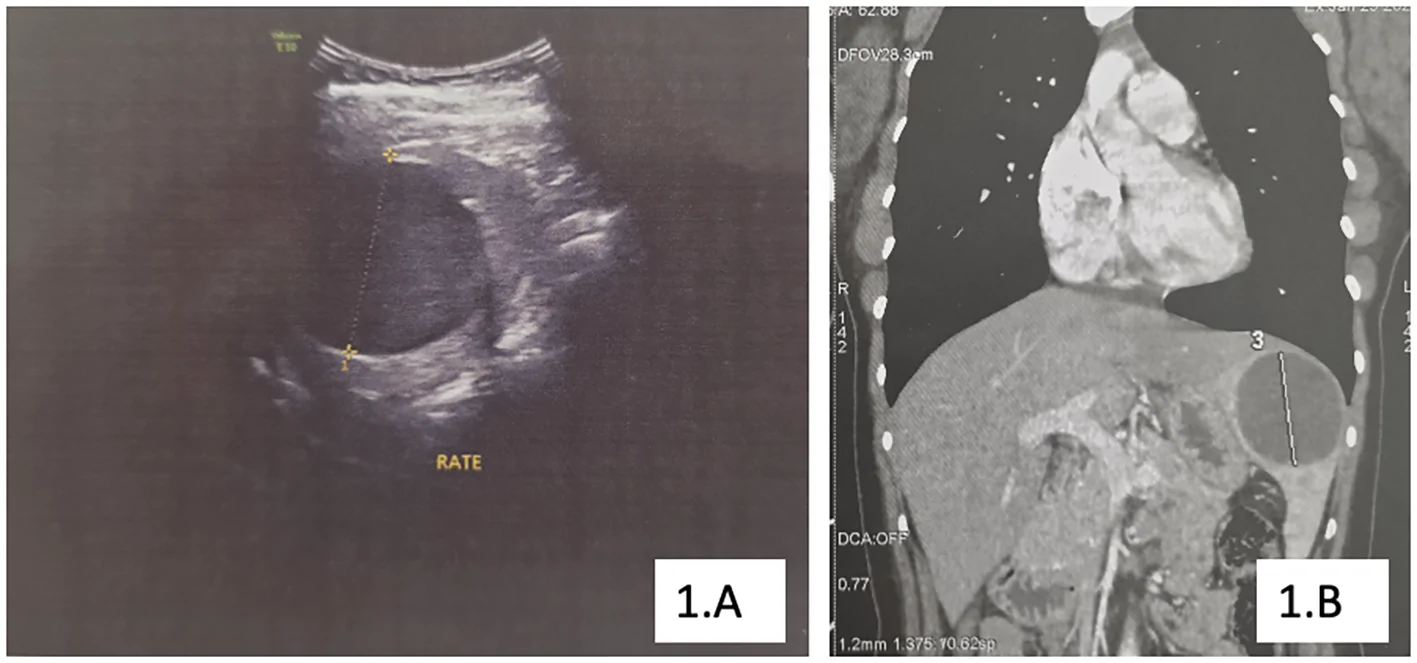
Preoperative imaging. (A) Abdominal ultrasound demonstrating a finely echogenic, well-circumscribed cystic lesion within the splenic parenchyma. (B) Contrast-enhanced abdominal CT showing a well-circumscribed, hypodense, homogeneous oval cystic mass measuring 50 × 57 × 56 mm at the upper pole of the spleen, with a thin, regular wall and a small peripheral calcification (arrow).
